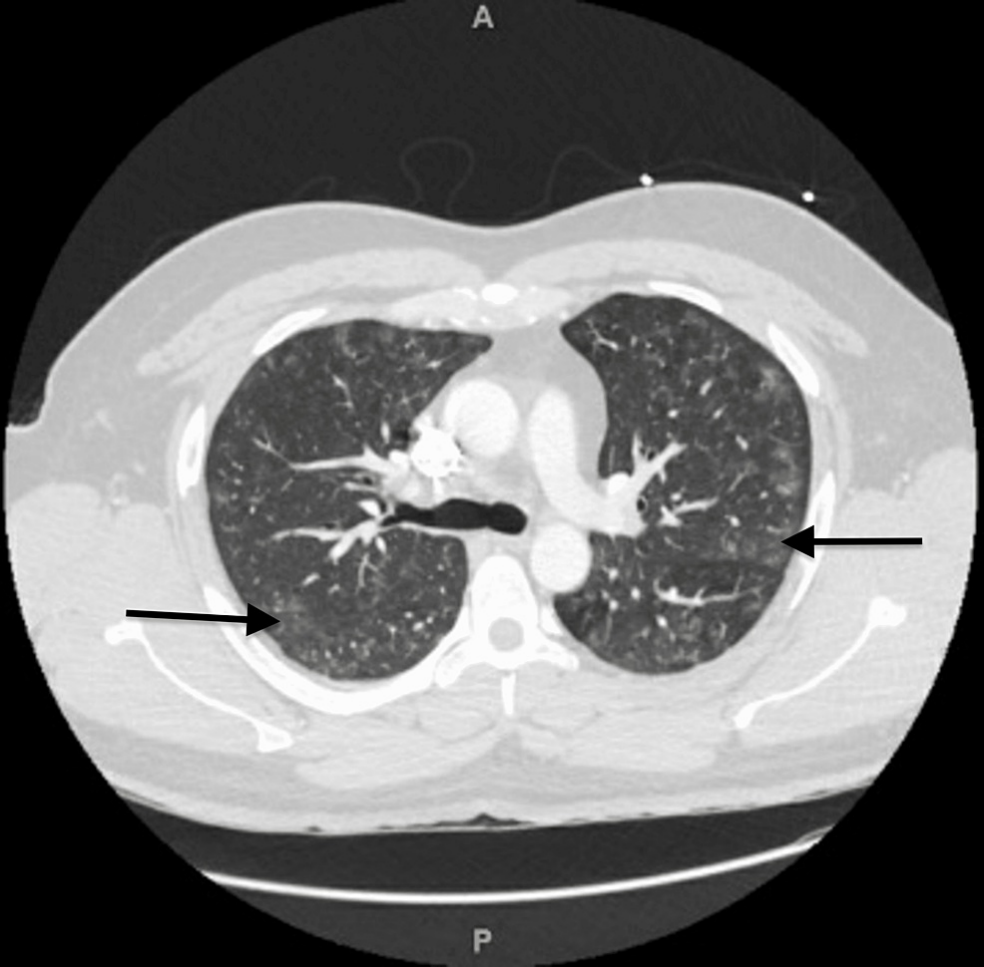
Computed tomography angiography (CTA) chest showing diffuse, bilateral ground glass lung opacities consistent with COVID-19 pneumonia.
